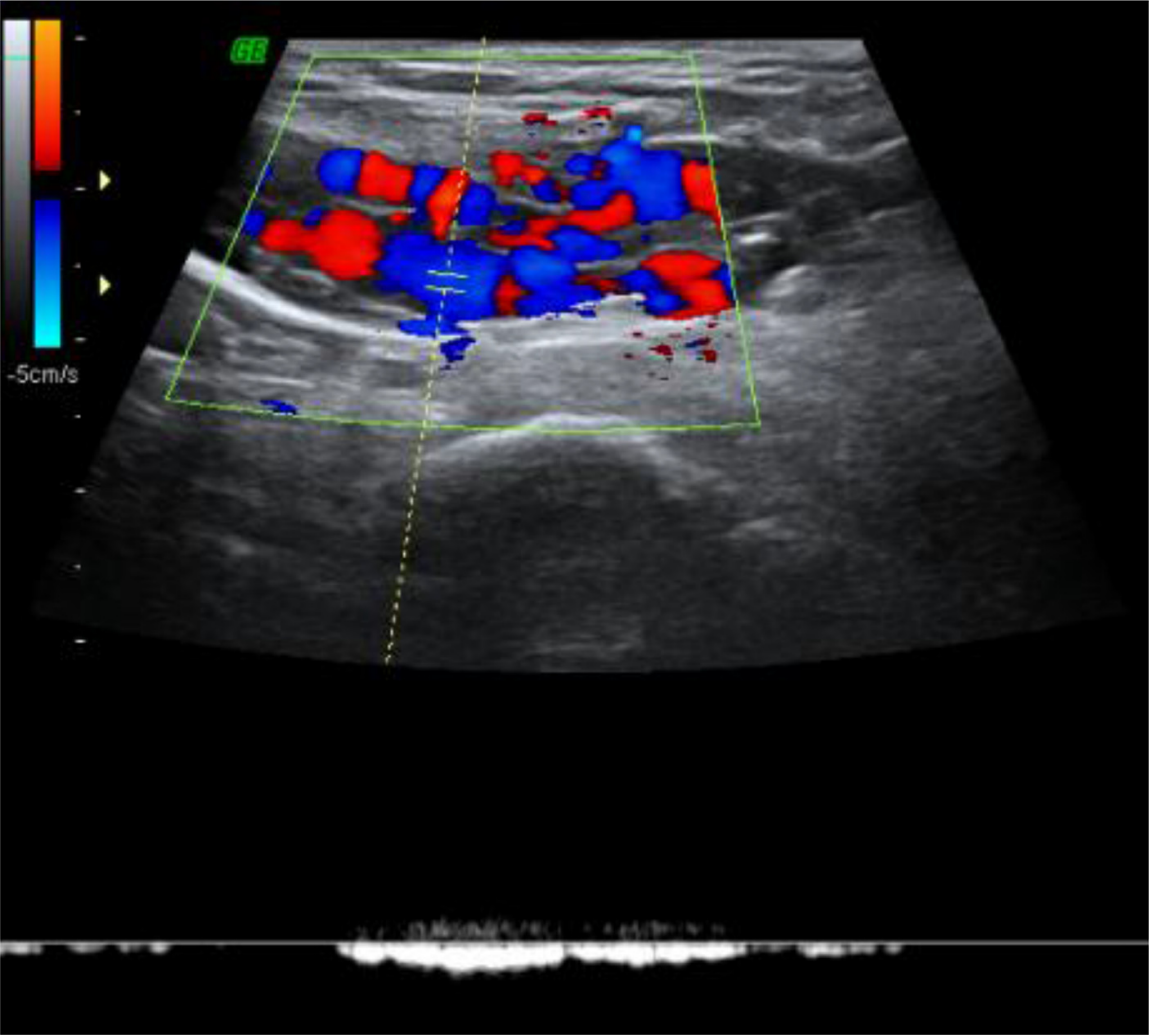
Pulse wave Doppler showing venous flow signal with reversal of flow on valsalva.
